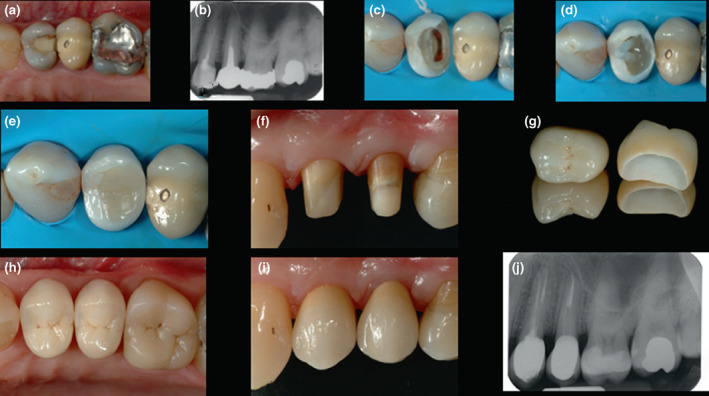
Zirconia crown (Lava; 3M ESPE AG) replacement 25 and crown placement 24 following root canal retreatment (a, b) preoperative occlusal view and long‐cone periapical radiograph (c) completed root canal retreatment and dentine surface preparation (d, e) pre‐endodontic build up, fibre post and core placement (f) completed full‐coverage crown preparations (g) crowns prior to cementation (h, i) finished restorations with postoperative occlusal and buccal views (j) follow‐up periapical radiograph at 10 years.
